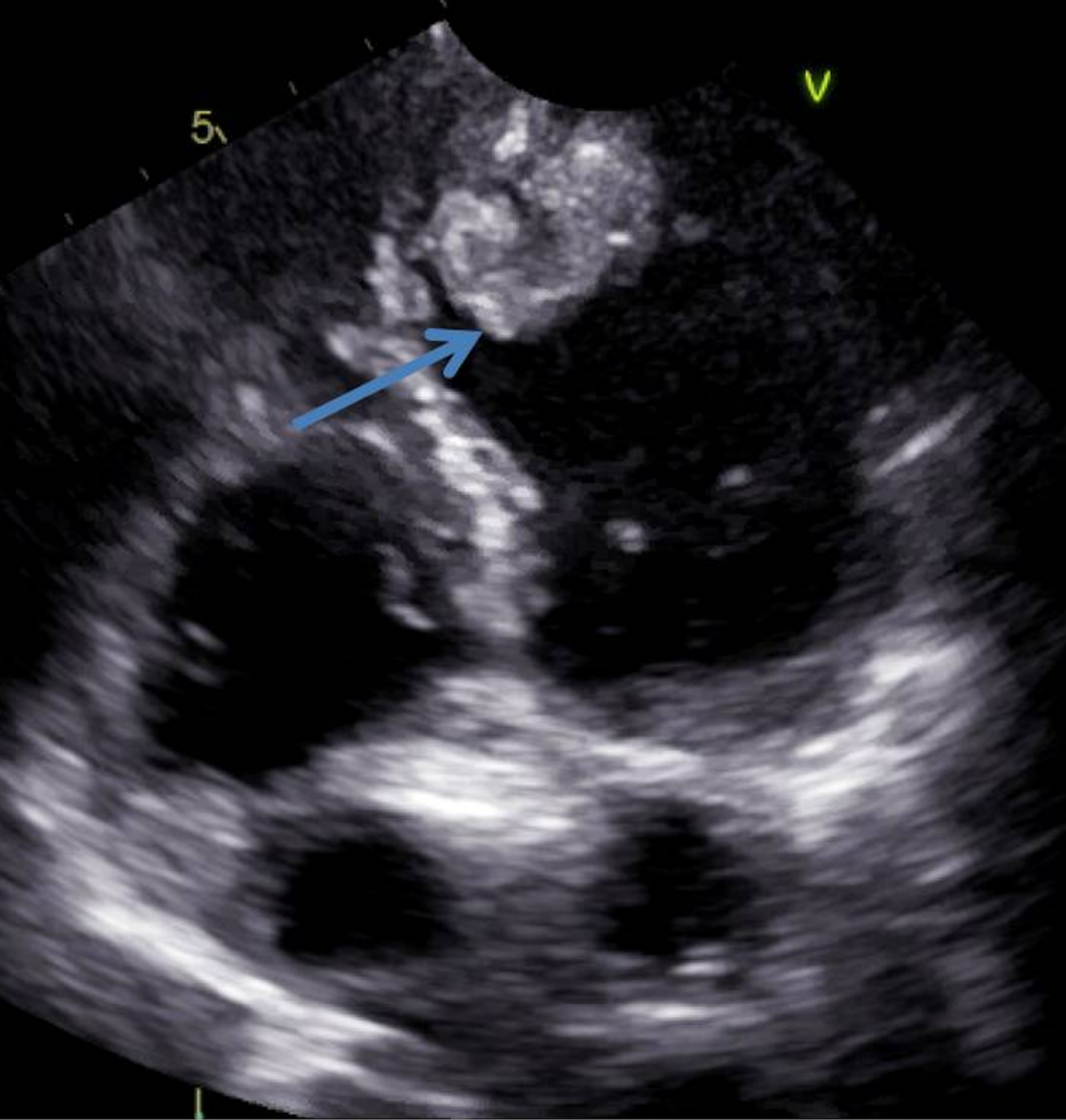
Echocardiogram -- apical four-chamber view demonstrating a heterogeneous left ventricular thrombus at the apex (arrow).
